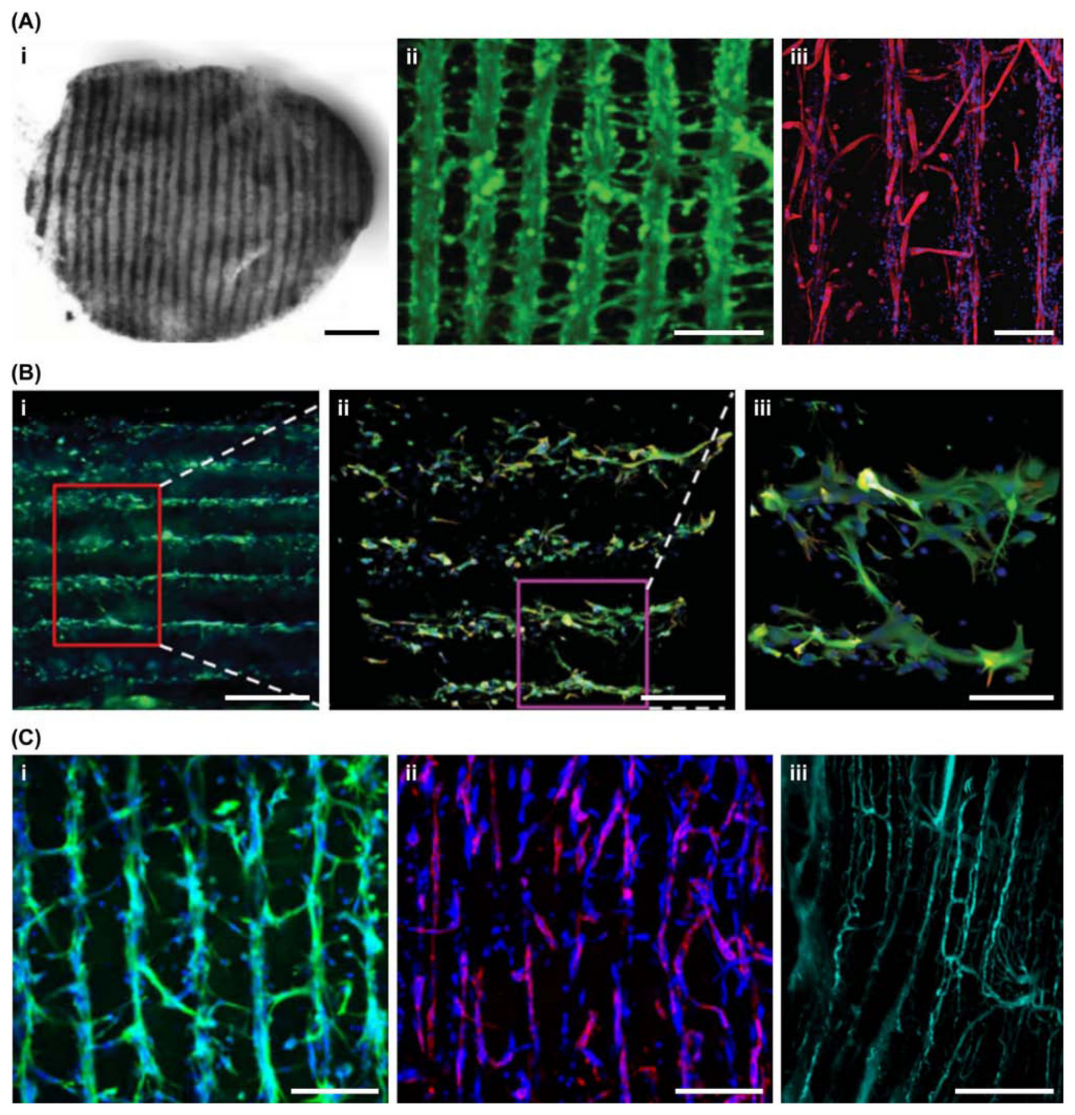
Acoustic Fields for Manipulating Cells. Ultrasound standing waves are particularly well suited for creating geometric
